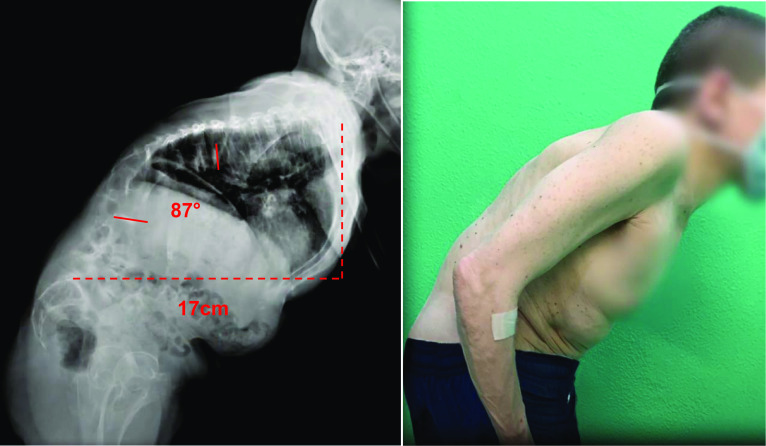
A 54-year-old female (case 1) with severe iatrogenic thoraco-lumbar hyperkyphosis (prior uninstrumented anterior and posterior thoraco-lumbar fusion): pre-operative radiographic and clinical presentation
